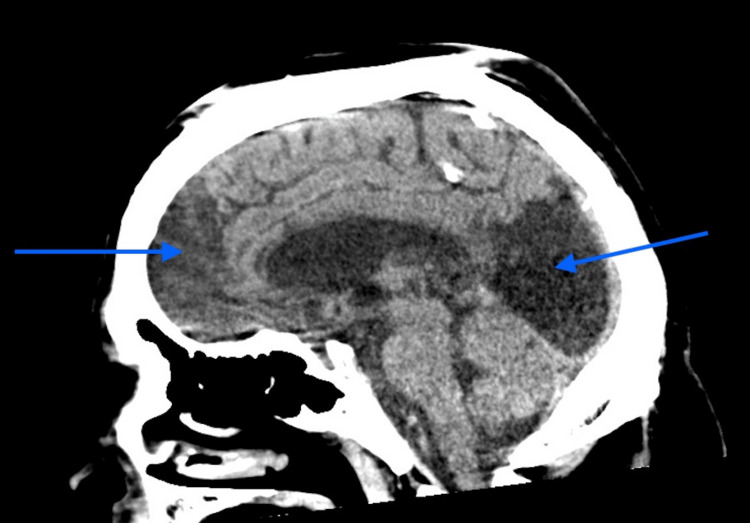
CT of the head - sagittal cut showing frontal and occipital lobe atrophy (blue arrows) CT: computed tomography
